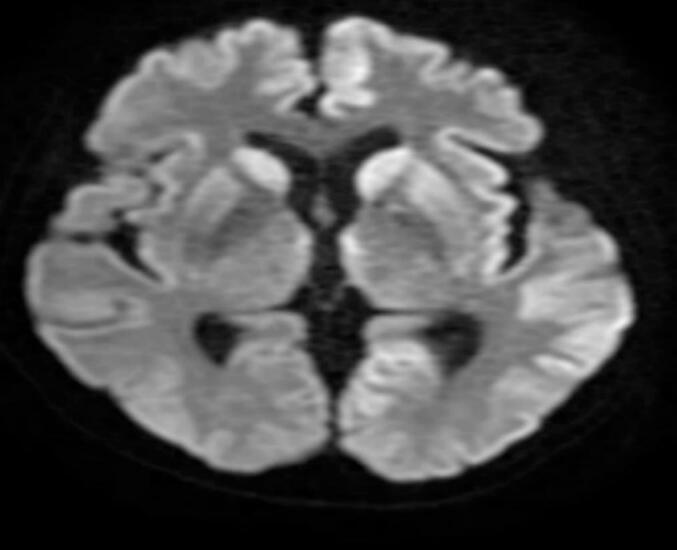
Diffusion-weighted MRI (DWI) shows hyperintensities in the bilateral caudate, putamen, and cortical regions in a Vietnamese patient with probably sporadic Cruetzfeld-Jakob disease.
